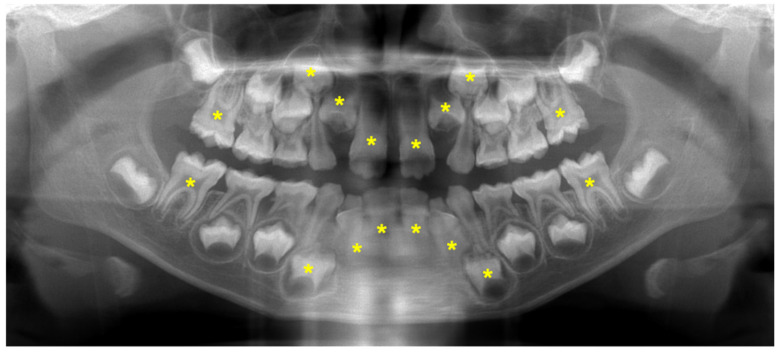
Orthopantomogram obtained at the first dental examination at 6 years 9 months of age. Generalized crown malformation and enamel defects were observed in both erupted and unerupted permanent teeth. Teeth affected by enamel hypoplasia are indicated by asterisks.
